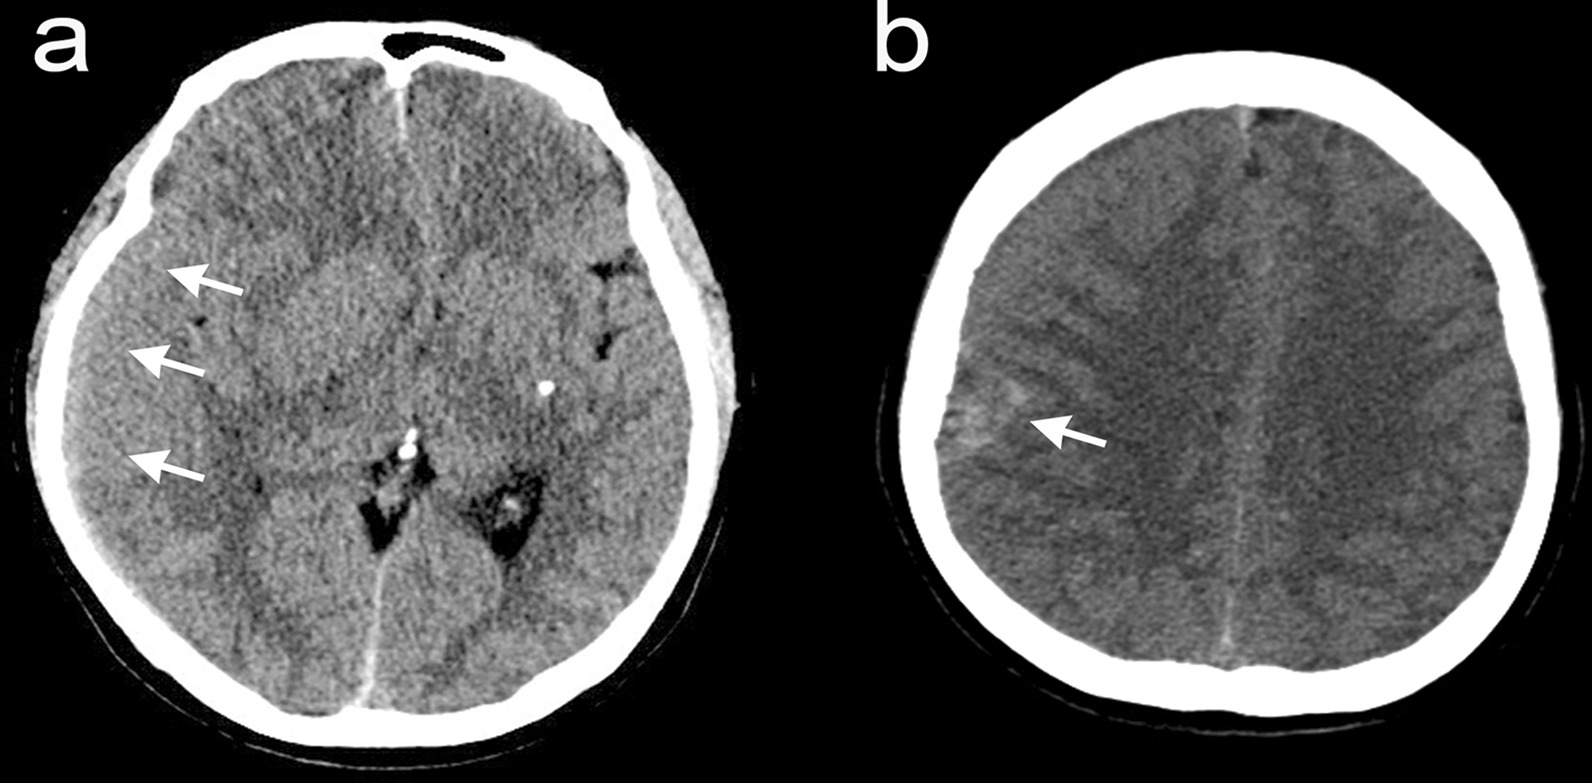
Preoperative axial computed tomography showing an acute subdural hematoma extending to the right fronto-temporo-parietal area (a, arrows) and a round-shaped, high-density mass on the right parietal convexity (b, arrow).
